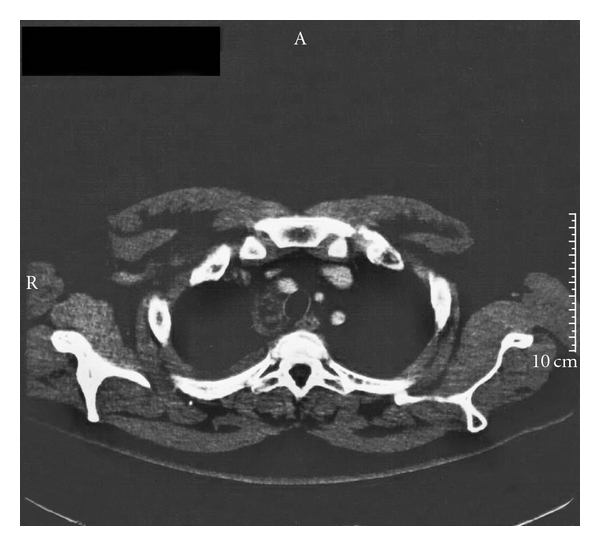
Axial enhanced CT-image of paratracheal right-sided mass and pleural effusion.
